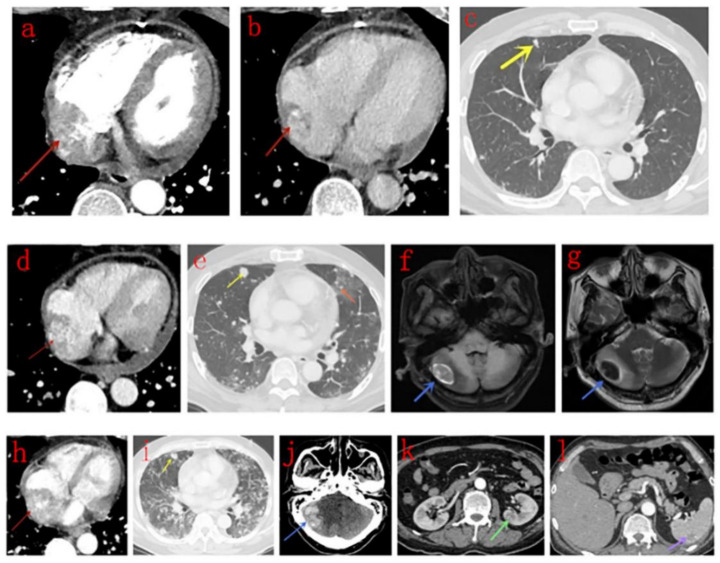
Male, 52 years old, right atrium angiosarcoma. (a–c): Contrast-enhanced computed tomography (CT) scans in the arterial (a) and venous (b) phase indicates a mass with heterogeneous centripetal enhancement (red arrow), and multiple solid nodules (c) are noticed in the lungs (yellow arrow). (d–g): 1.5 months later, pericardial effusion appears (d), metastases (yellow arrow) in the lungs are more extensive, halo sign appears (e, orange arrow). Metastasis in the right side of the cerebellum (blue arrow) presents as inhomogeneous hyperintensity on T1WI (f) and hypo-intensity on T2WI (g) with surrounding edema. (h–l): Another month later, the mass (red arrow) becomes larger, and the adjacent pericardium invasion is more extensive (h), and metastases in the lungs are much more extensive (i). The metastasis in the right side of the cerebellum (blue arrow) is larger (j). Metastases in the kidney (k, green arrow) and spleen (l, purple arrow) appear.
